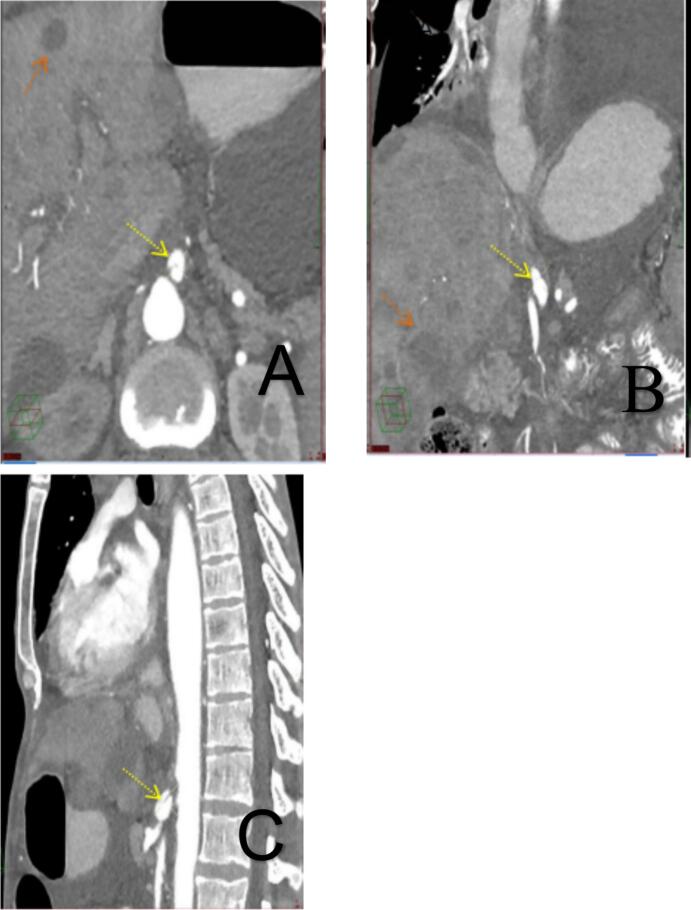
Axial (A), Coronal (B), Sagittal (C) CT showing dissecting flap (yellow arrow). Abdominal CT scan with IV and oral contrast (shown in figure above) - axial, coronal and sagittal views (A, B, C respectively), arterial phase: focal aneurysmal dilatation of the celiac trunk with evidence of internal dissecting flap (yellow arrow) and liver shows multiple metastatic lesions (organ yellow A, B). (For interpretation of the references to color in this figure legend, the reader is referred to the web version of this article.)
